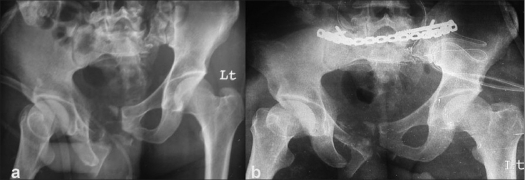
Preoperative (a) and postoperative (b) radiographs showing a vertical shear type of pelvic disruption with a zone 2 fracture of the sacrum fixed with a posterior percutaneous reconstruction plate and lag screw fixation. Note that the vertical shear component of the disruption has been addressed
